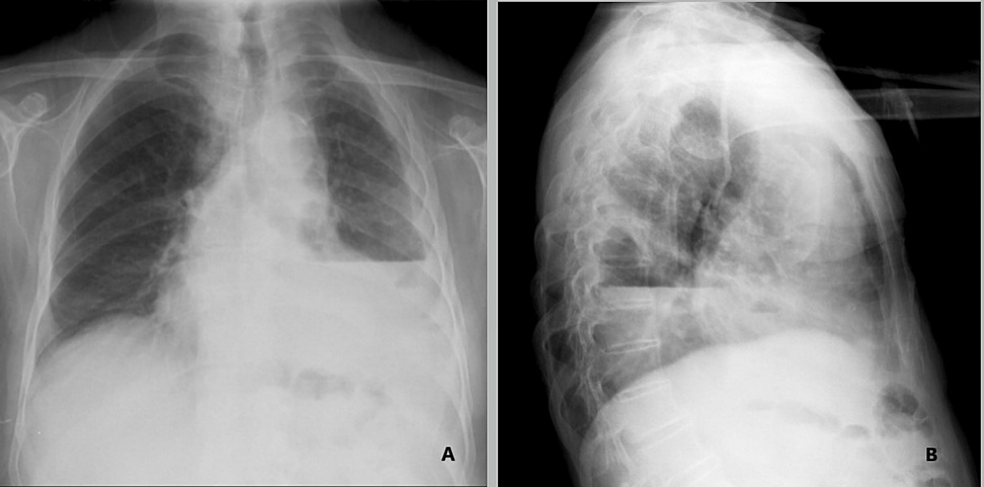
Chest x-ray showing pleural effusion with air-fluid level. (A) Anteroposterior view of the air-fluid level extending all the way to the chest wall. (B) Lateral view of the air-fluid level. Open access. Creative commons license. Case courtesy of Assoc Prof Frank Gaillard, Radiopaedia.org, rID: 15390
